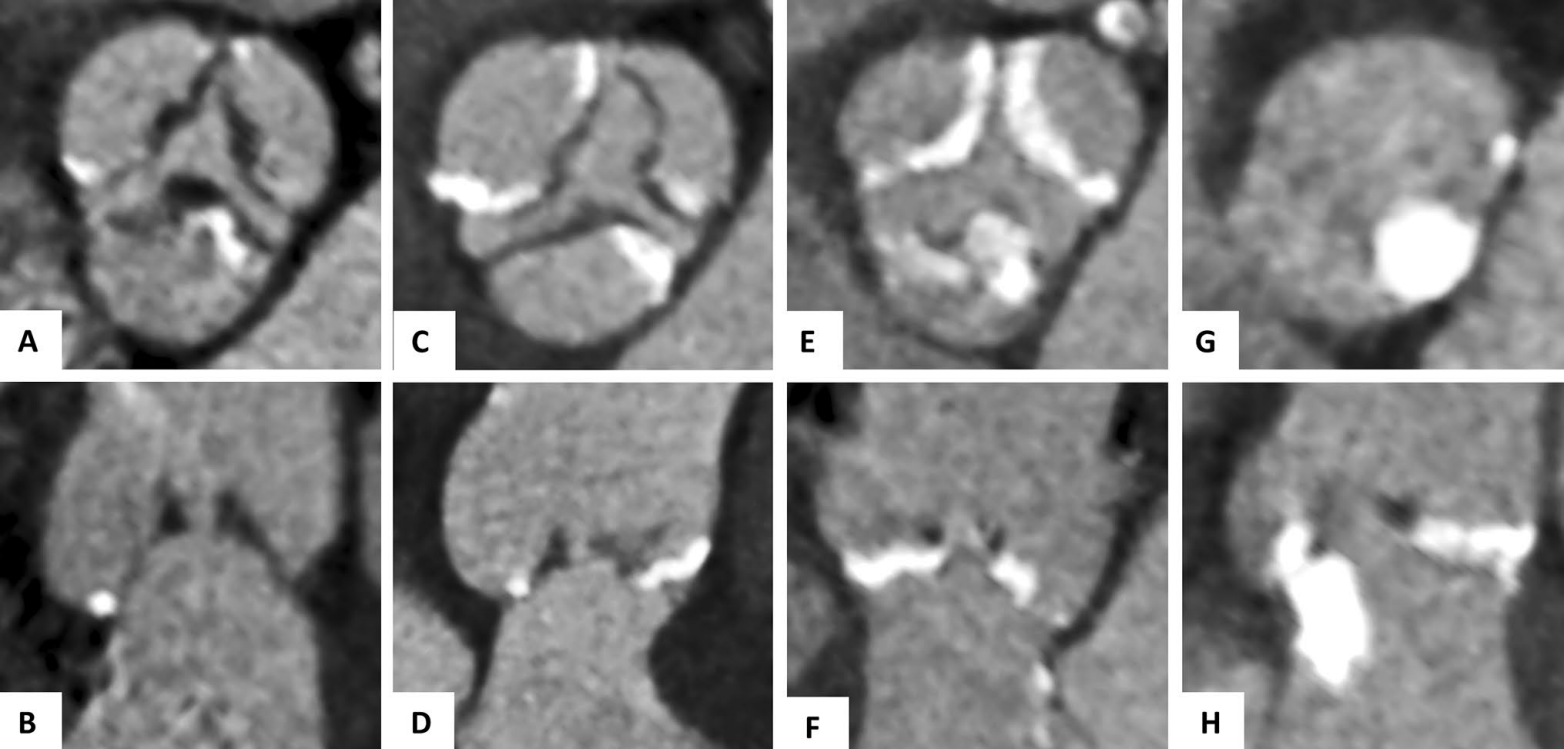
Semiquantitatitve calcification assessment of the device landing zone. Pre-TAVI contrast-enhanced CTA axial and sagittal oblique reconstruction for visual calcification assessment with examples for mild (a, b), moderate (c, d) or severe (e, f) calcification of the cups regions and a severe calcification of the region below the annulus (g, h)
